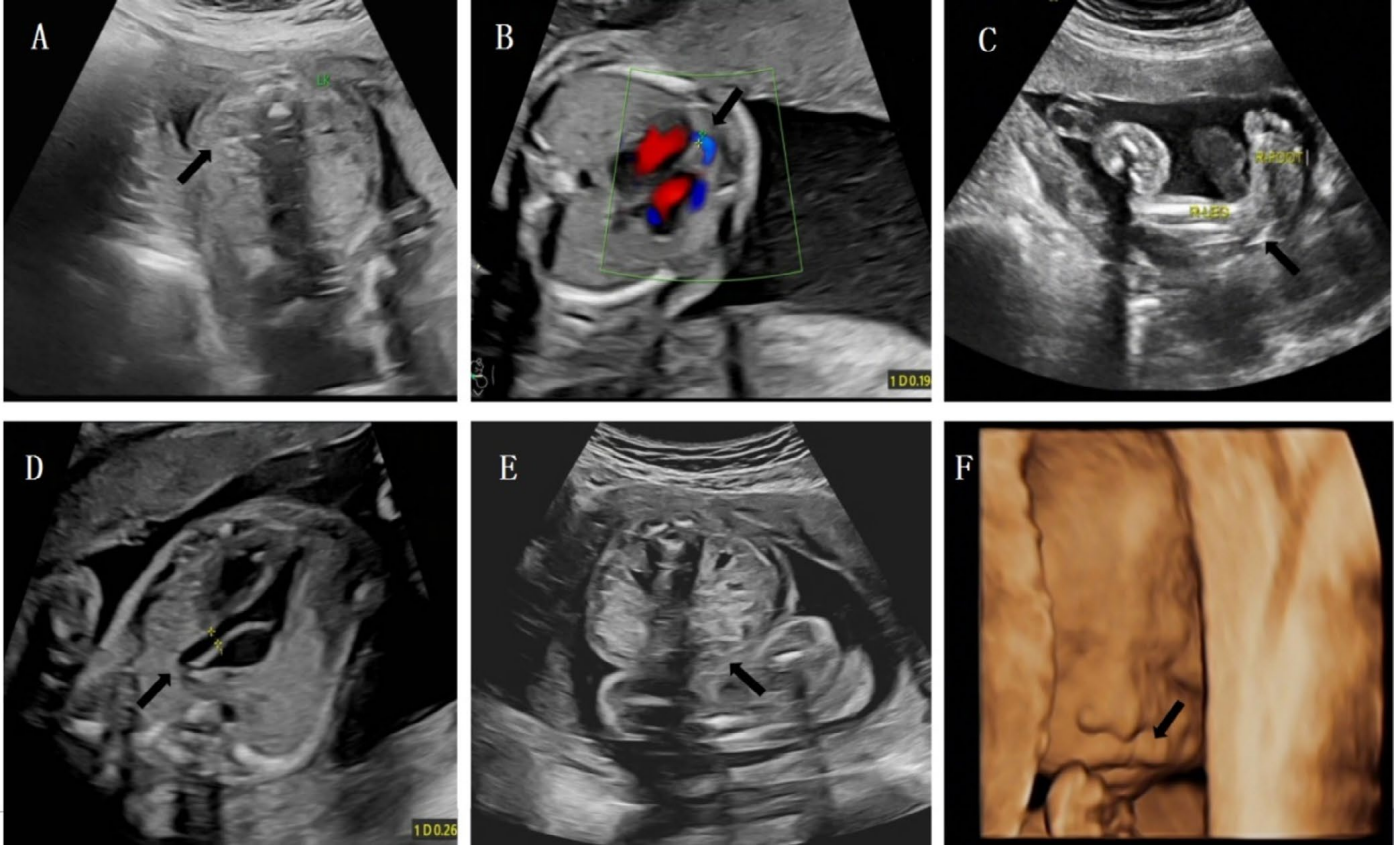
Ultrasound findings of Case 2 (A–C) and Case 14 (D–F) showed multiple structural malformations.
